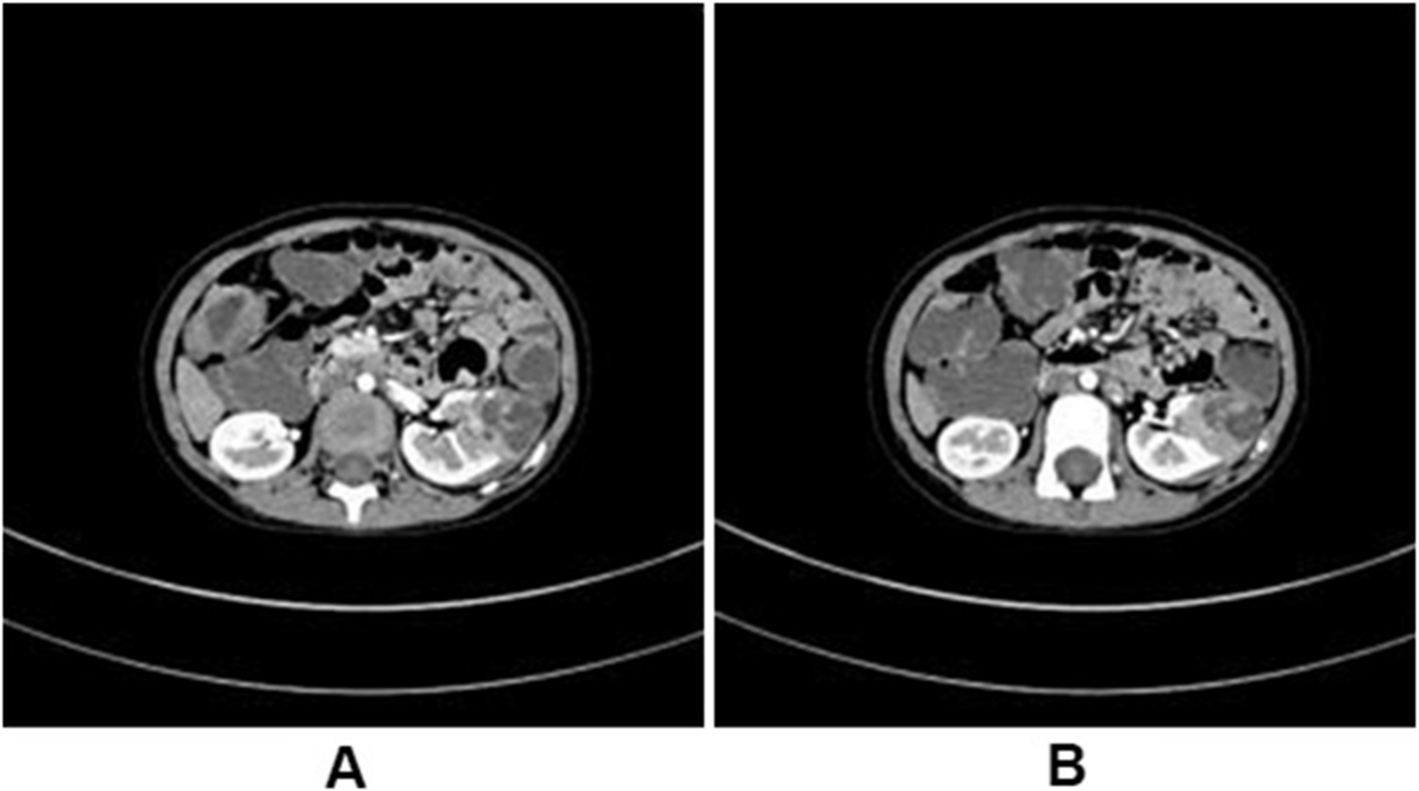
Different sections of the computed tomography scan after chemotherapy revealed an approximately 3.1 × 3.2 × 4.2 cm irregular mass in the left renal parenchyma, with the shadows of irregular soft-tissue masses
